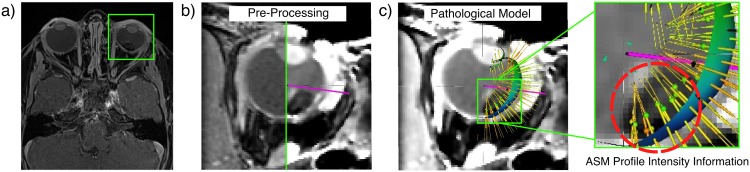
Learning a Pathological Eye Model (PM). We follow the steps in [15] to (a) automatically detect the eye in the 3D MRI, followed by a set of b) image pre-processing techniques to learn information of pathological and healthy structures jointly using c) intensity profiles containing pathological information.
